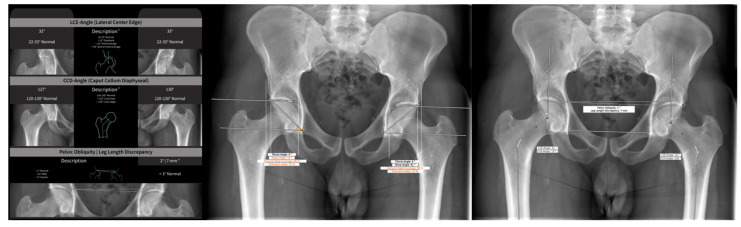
AI software (HIPPO™) report of an AP radiograph of the pelvis providing fully automated measurements.
